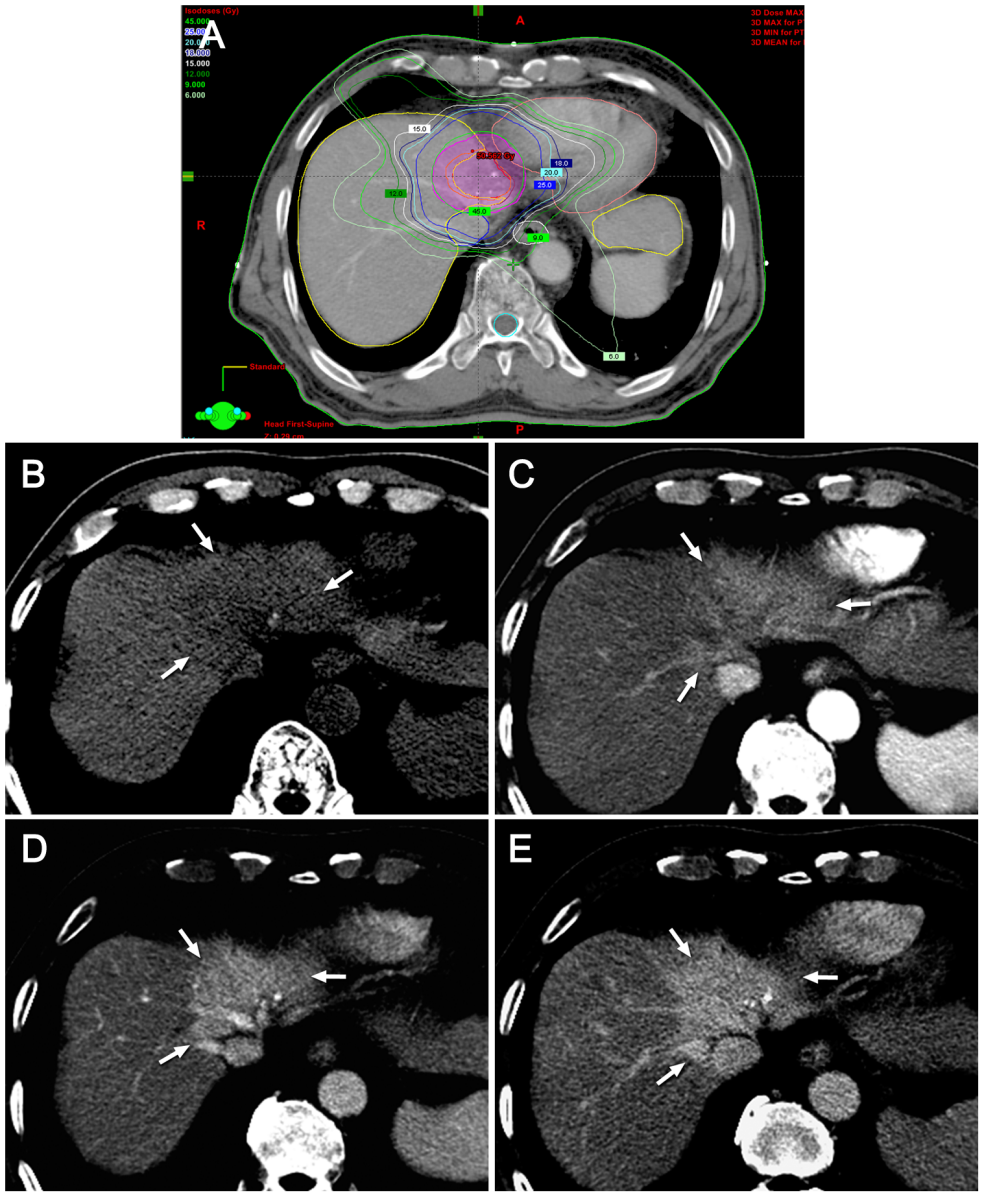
Typical hepatic attenuation difference seen on six months after SBRT. (A) The isodose curve for SBRT depicts the planning target volume (PTV, purple circle) as well as the gross tumor volume (red circle) for the treatment of hepatocelluar carcinoma in left lobe of the liver. The total radiation dose for this patient was 45 Gy. The irradiated hepatic parenchyma is delineated corresponding to the region inside the 15-Gy isodose line but outside the PTV boundary. (B-E) Irradiated hepatic parenchyma appears as low attenuation (arrows) on noncontrast CT (B) and as high attenuation on the arterial (C), portal (D), and delayed (E) phase, respectively.
